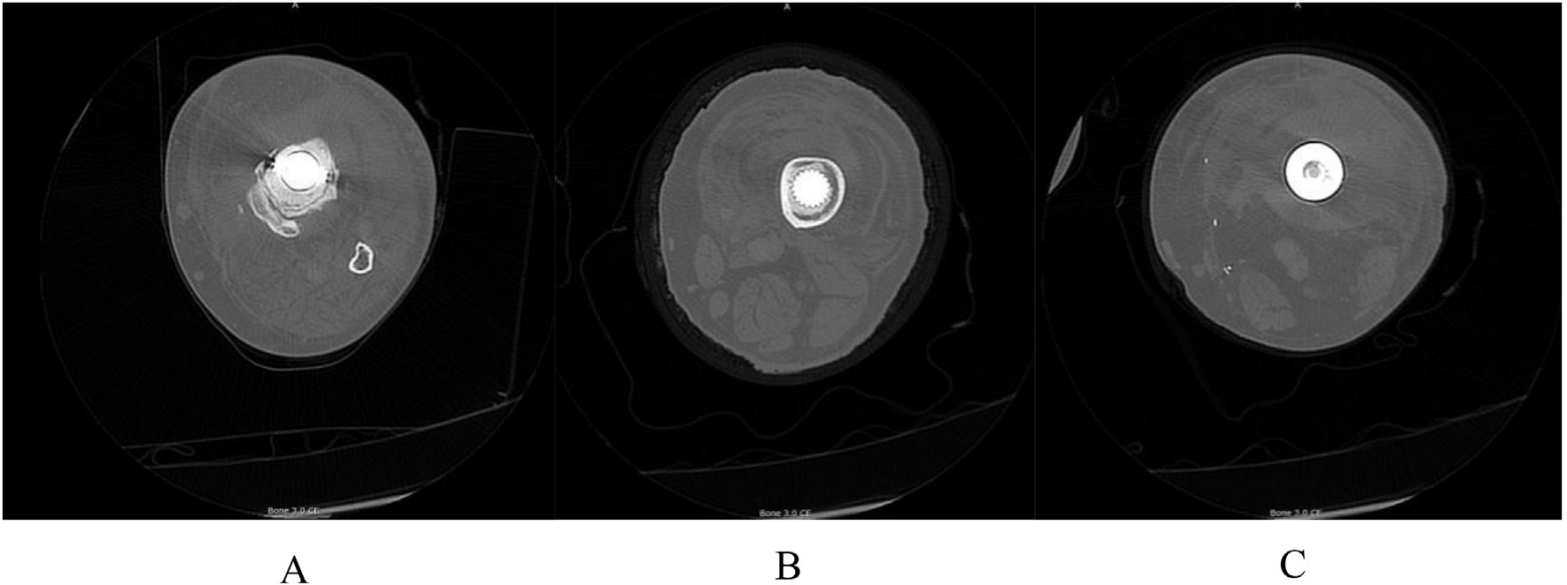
A CT scan provides a three-dimensional look inside the bone, muscle, and fat. Transaxial cuts from distal to proximal sections along the leg (images A-C) are from the same patient. A. Demonstrates increased reactivity around the tibial component. B. Demonstrates a well-fixed femoral component with synovial effusion. C. Demonstrates large effusion adjacent to the femoral component as well as reactive soft tissue.
